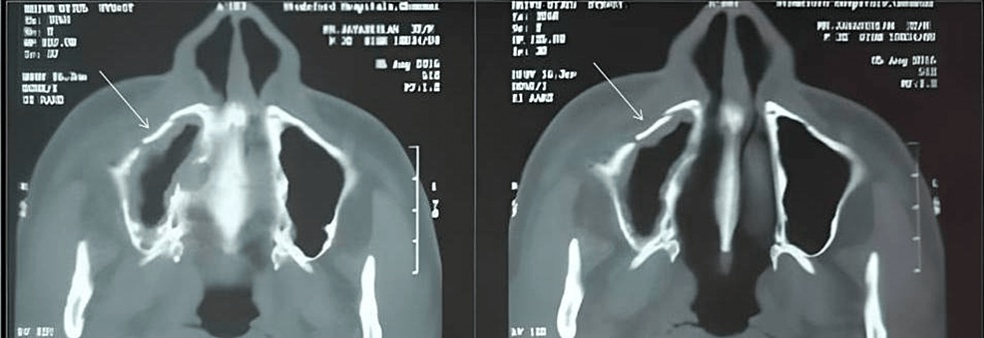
Reduction of fractured segments, as seen in the axial section of the CT scan (postoperative)
